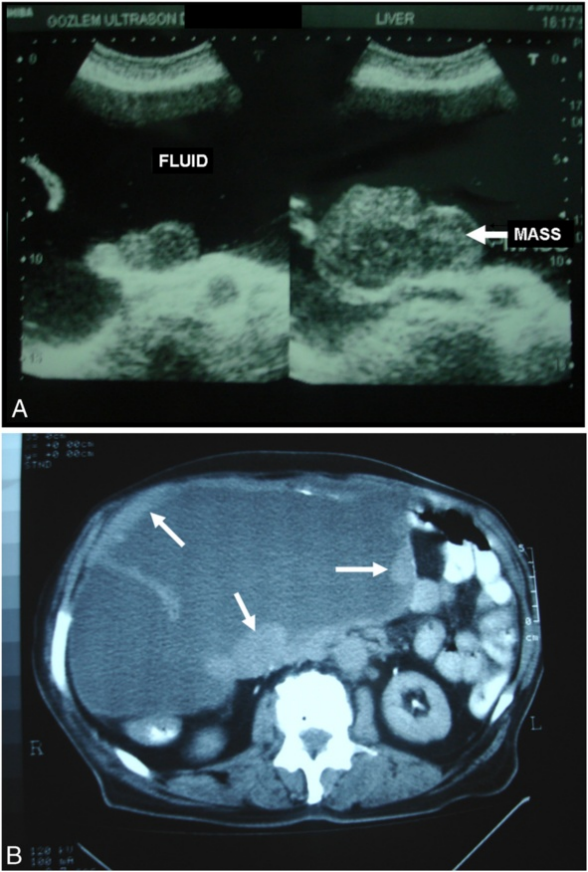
(A) An abdominal USG revealed multiple heterogeneous masses (*arrows*) in one large cystic cavity. (B) An abdominal CT revealed a huge polycystic tumor, almost completely filling the abdomen, with a part of the thickened wall (*arrows*).
